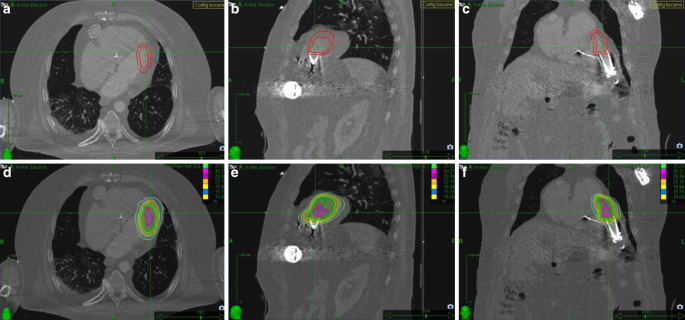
Case I: clinical and planning target volumes in axial (a), sagittal (b) and coronal plane (c) and dose distribution of radiosurgery treatment plan in corresponding levels of planning computed tomography in axial (d), sagittal (e), and coronal views (f). Dose distribution is displayed to the 15 Gy isodose. The *green line* displays the 25 Gy isodose
